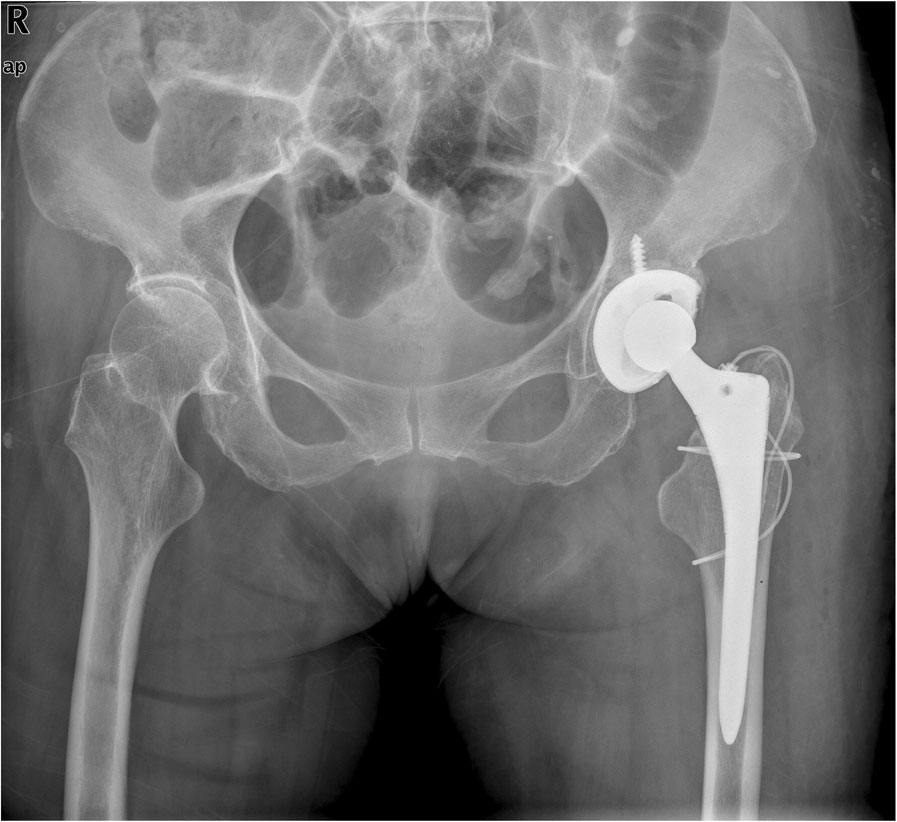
Anteroposterior radiograph 2 years after ORIF showed reduction and fixation of the fracture, the fracture healed well, and the stem was stabilized
